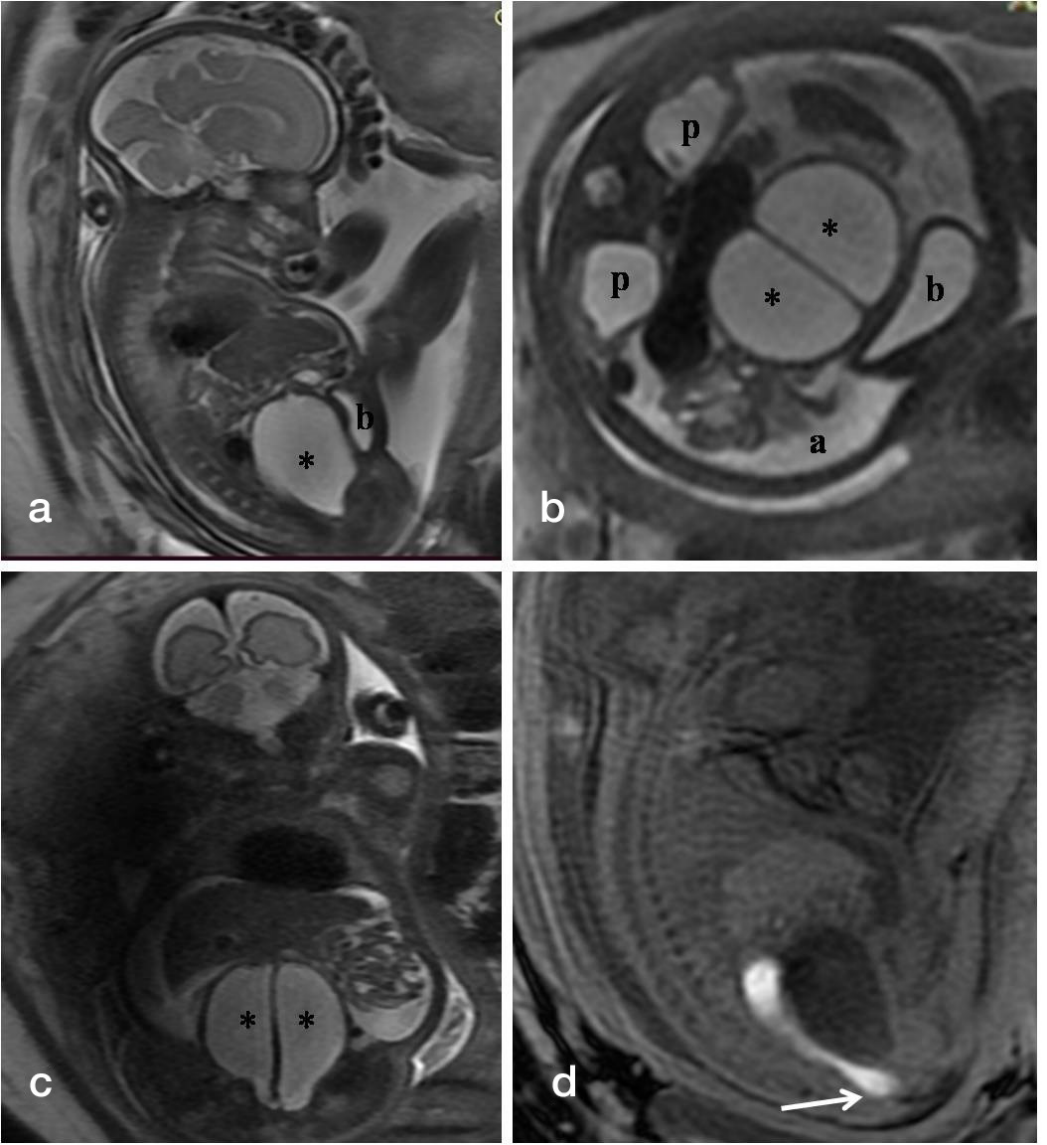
(a-d). T_2_-weighted fetal MR images in sagittal (a), axial (b), and coronal (c) planes show the distended vagina (*) with central linear hypointense vertical septum displacing the bladder (b) anteriorly against the abdominal wall, bilateral renal pelvic dilatation (p) and fetal ascites (a). Sagittal T_1_-weighted MR image (d) reveals hyperintense meconium within non-dilated rectum seen reaching upto the perineum.
